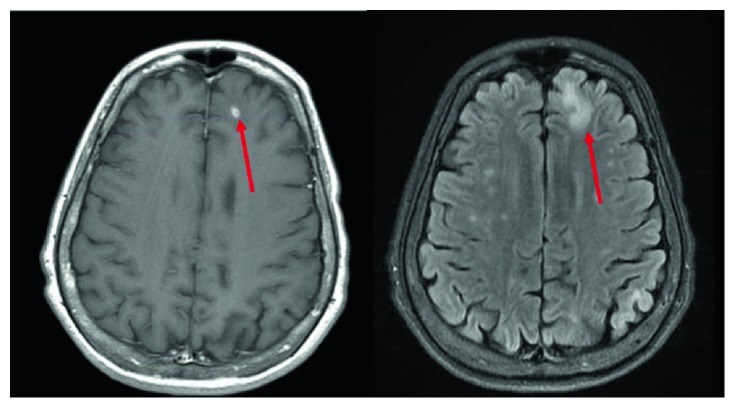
Axial T1 with contrast (left) and axial T2 FLAIR (right) magnetic resonance imaging
